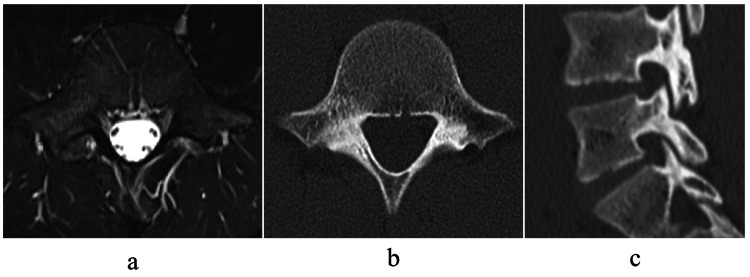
Imaging at 3 months after first examination Three months after the first examination, the signal change on magnetic resonance images (MRI) disappeared (a), and bone union was achieved with no obvious worsening of the fracture on computed tomography (CT) (b, c).
